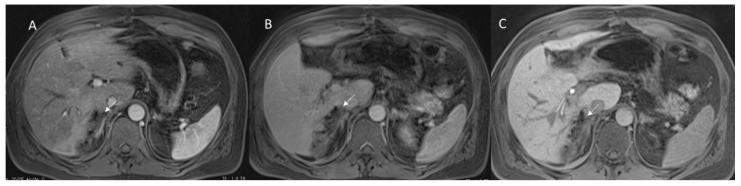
Hepatic abscess in resected cholangiocarcinoma on VI hepatic segment, evaluated with MRI. Arrow shows air artifacts within the collection and hyperenhancement of hepatic parenchymal in arterial phase (A) of contrast study that disappears in portal (B) and hepatobiliary (C) phase of contrast study.
